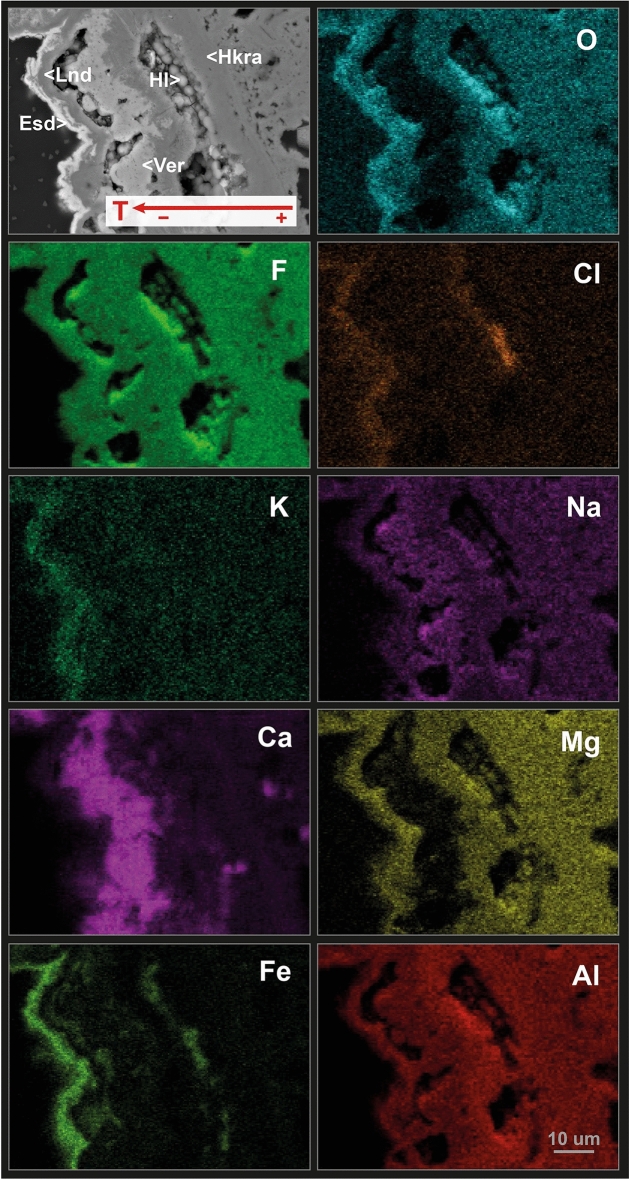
Wavelength-dispersive X-ray maps of representative elements of the typical fluoride-chloride assemblage from the Tajogaite proximal fumaroles: erytrosiderite (Esd) leonardsenite (Lnd), verneite (Ver), halite (Hl) and hydrokenoralstonite (Hkra).
